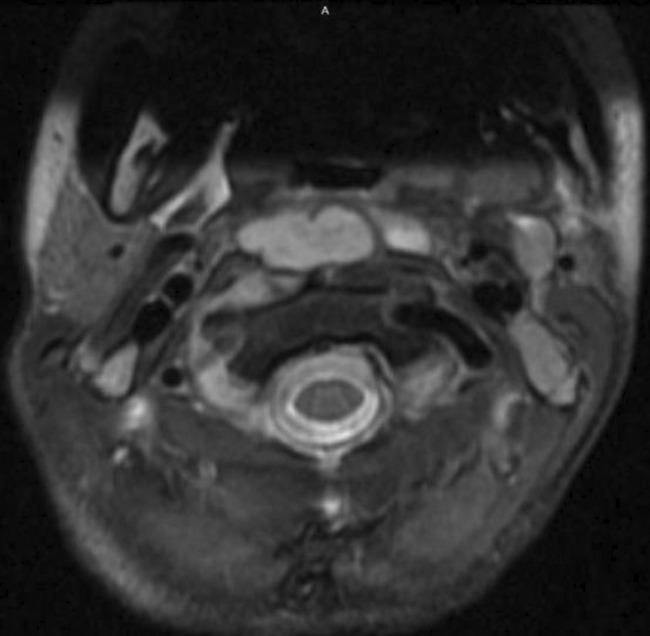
Cervical spine MRI (axial view) well‐defined retropharyngeal/danger space collection measuring 3.8 × 1.4 × 3.1 cm
